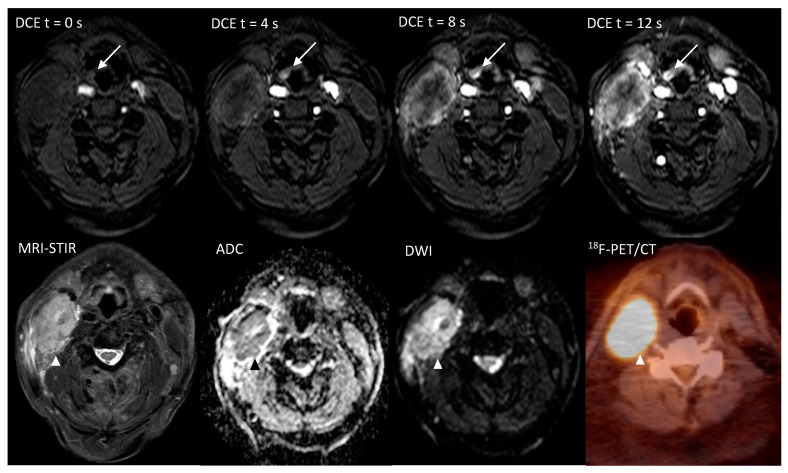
Imaging of a 71-year-old patient, with a hypopharyngeal (epiglottal) primary tumor detected only on the ultrafast-DCE imaging (arrow), whereas DWI and ^18^F-FDG-PET/CT did not detect the lesion. The overt malignant lymph node metastasis is marked with an arrowhead (Δ).
